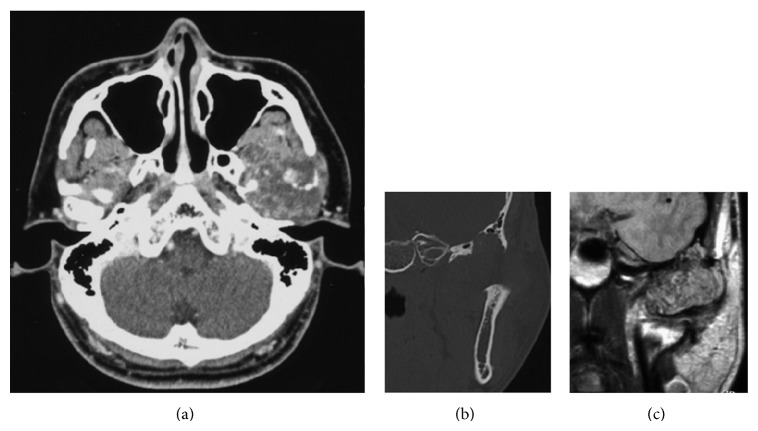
(a) Low-enhanced tumor around the condyle head in contrast-enhanced CT scan; (b) infratemporal fossa erosion and middle cranial invasion of tumor in coronal view of CT scan; (c) tumor expansion close to dura in enhanced MRI.
